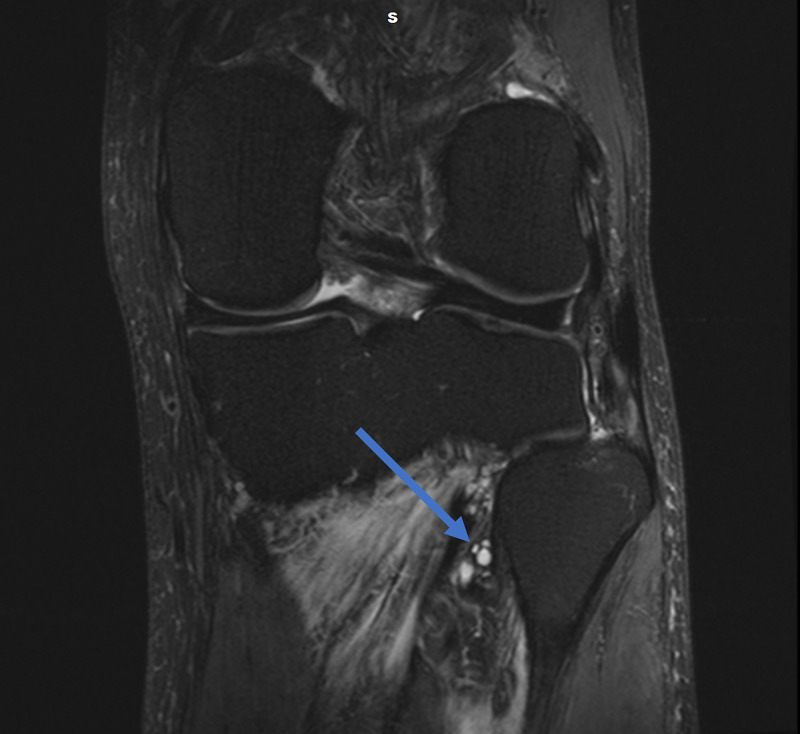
Coronal T2-weighted TSE fat-saturated image demonstrating cysts connecting to the posteromedial superior tibiofibular joint TSE, turbo spin echo
